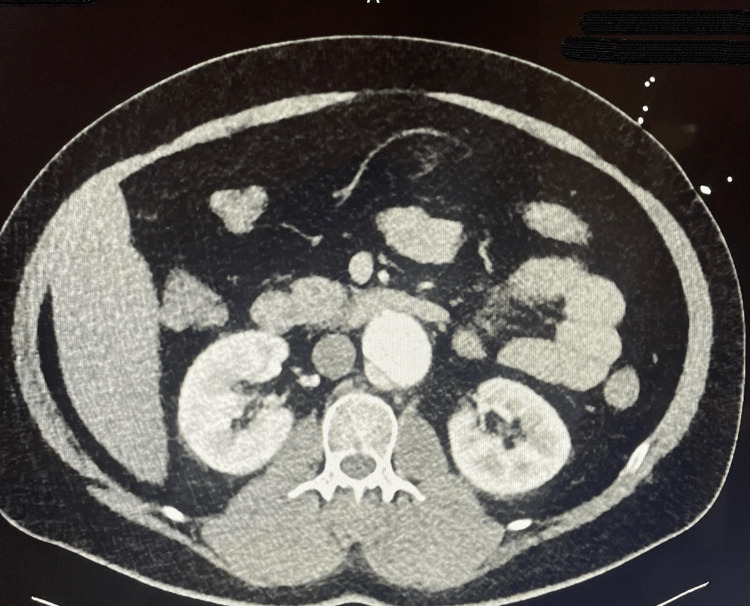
CT angiography of the abdomen showing that a second dissection flap was noted distal to the superior mesenteric artery.
